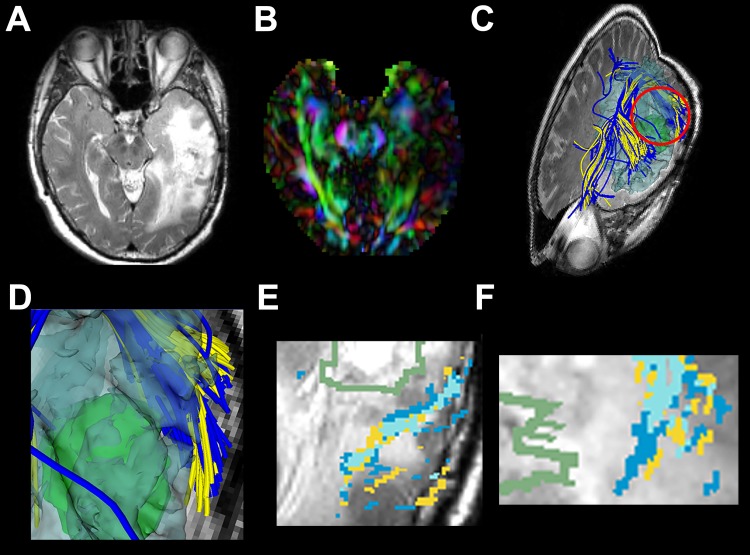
Images from P11 with a left temporal GBM. (a) T2-weighted image shows a temporal tumor with extensive edema around the lesion. (b) Directionally encoded color FA map shows reduced anisotropy in AF. (c) The AF is reconstructed by the UKFt (yellow tracts) and UKFt+FW (blue tracts) methods. (d) The magnified red circle (in c) area shows a slight difference in fibers traced using the UKFt+FW vs the UKFt method near the tumor (green surface model). (e) Magnified axial view of a T2-weighted image with overlaid label maps showing fibers reconstructed by the UKFt (yellow) and UKFt+FW (dark blue) methods and where they overlap (light blue) near the tumor (green outlined). (f) Magnified sagittal view of a T2-weighted image with overlaid label maps showing fibers reconstructed by the UKFt and UKFt+FW methods and where they overlap near the tumor.
